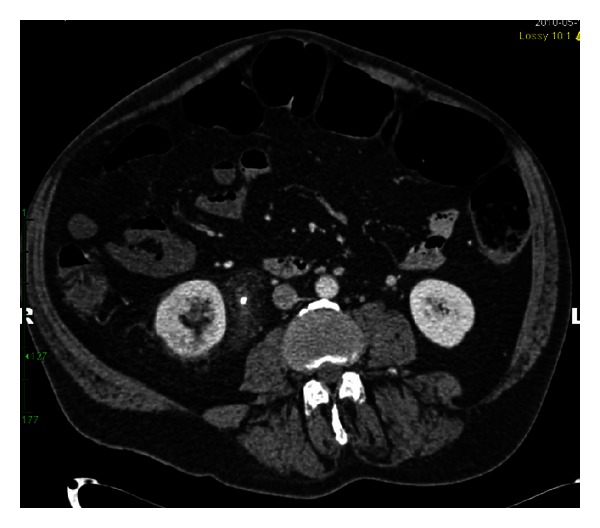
CT abdomen with contrast demonstrates a wedge-shaped segment of low attenuation in the posterior, lower pole of the right kidney consistent with pyelonephritis. Please note the retained right ureteral stent and periureteral stranding.
